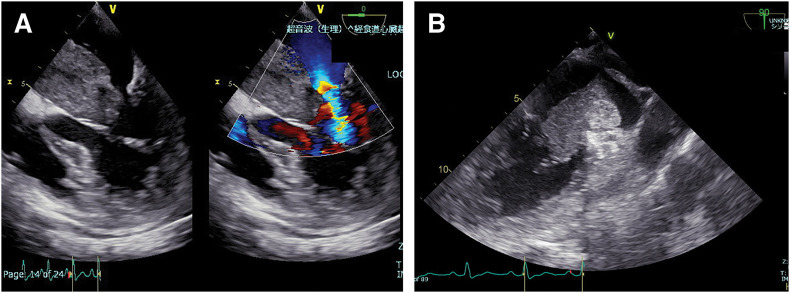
Transesophageal Echocardiography (A) Mobile LA mass prolapsing into the mitral orifice. (B) Imminent ball-valve obstruction during transient fluid reduction. LA, left atrial
